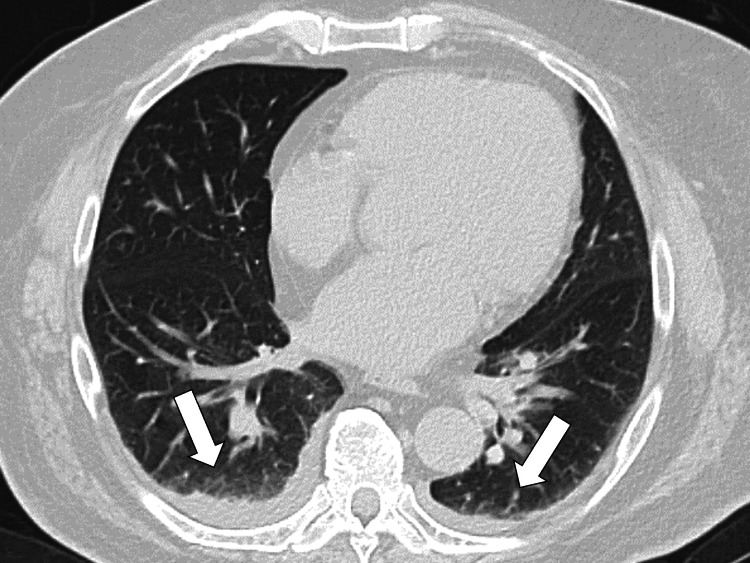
The chest computed tomography scan showed bilateral pleural effusion (white arrows).
